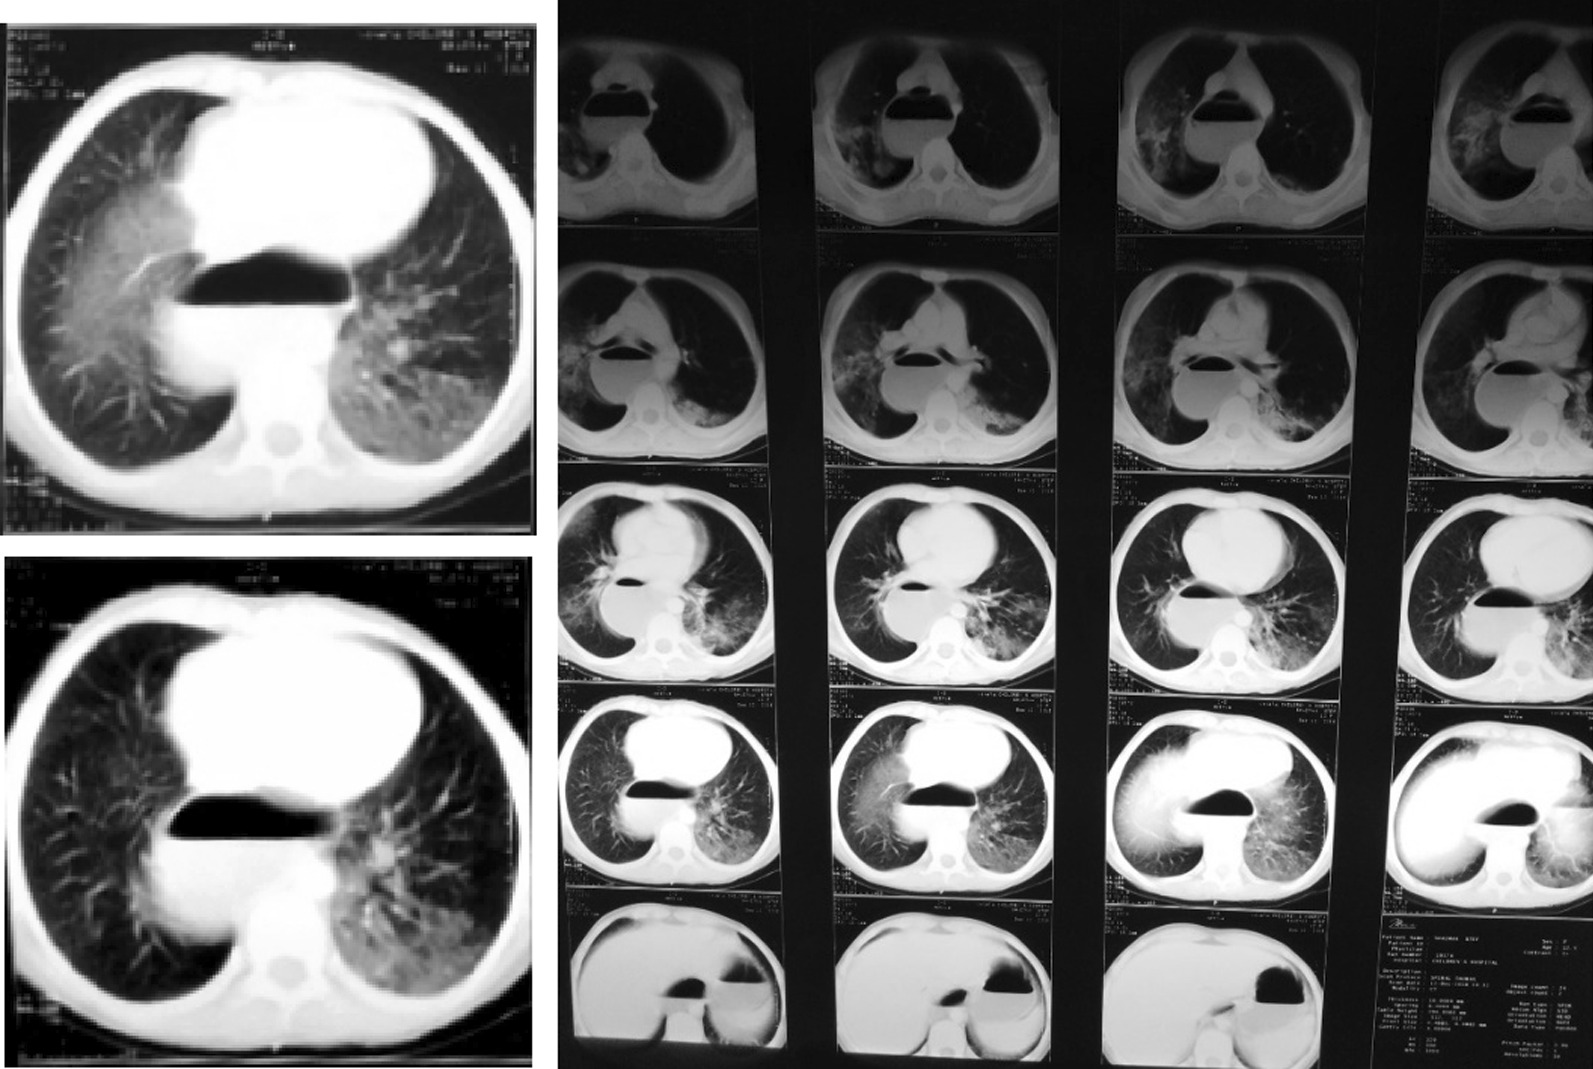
Computed tomography (CT) scan of the thorax showed massive dilation of the esophagus and bilateral pulmonary infiltration.
